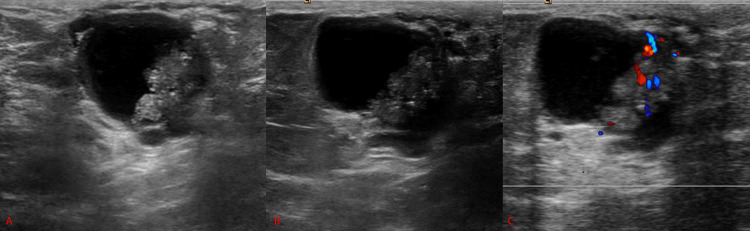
Longitudinal (A) and transverse (B) sonographic images of the right breast demonstrates a complex mass with solid and cystic components. Doppler ultrasound (C) demonstrates increased vascular flow in the solid component.
